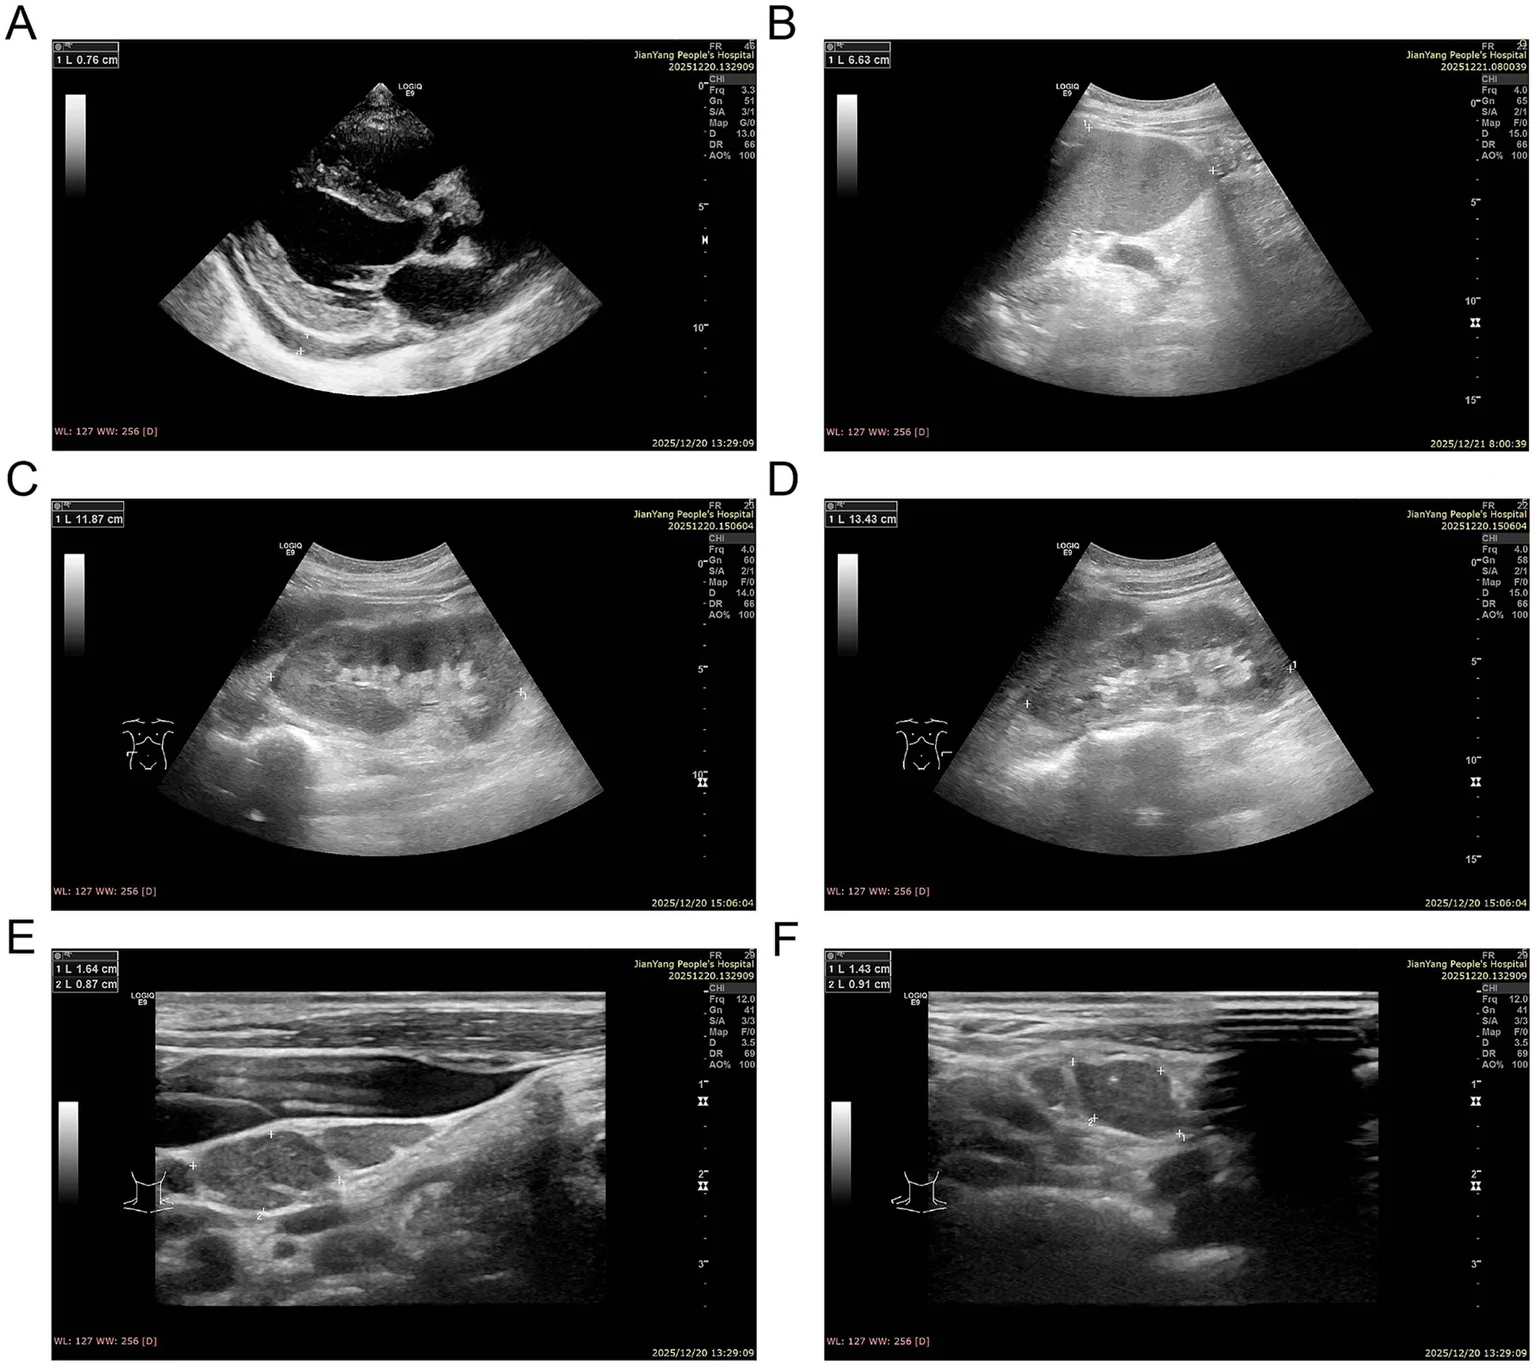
Ultrasound findings. (A) Echocardiography demonstrating pericardial effusion. (B) Abdominal ultrasound showing splenomegaly. (C,D) Renal ultrasound revealing bilateral kidney enlargement. (E,F) Cervical ultrasound showing bilateral enlarged cervical lymph nodes with cortical thickening.
